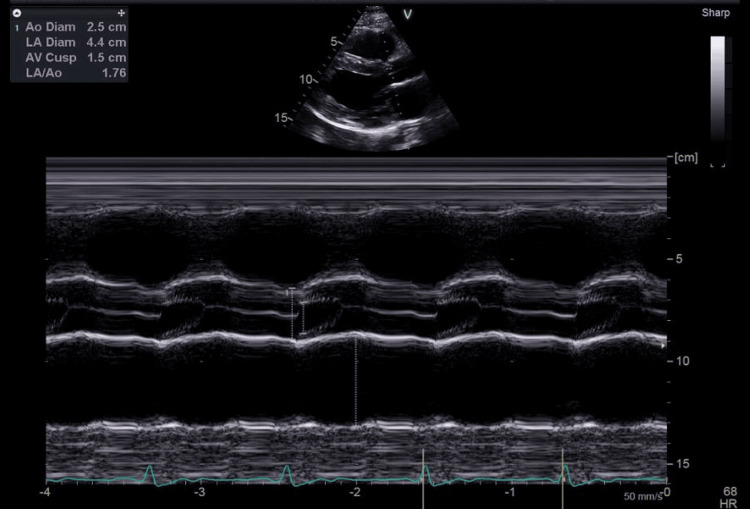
Parasternal long-axis (PLAX) view with M-mode through the left ventricle on echocardiogram
